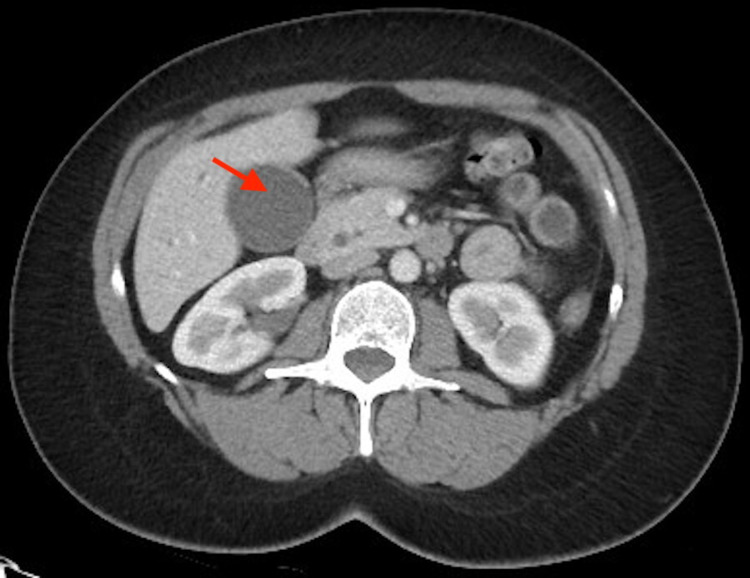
CT angiography scan demonstrating hydropic gallbladder on the left (red arrow)
